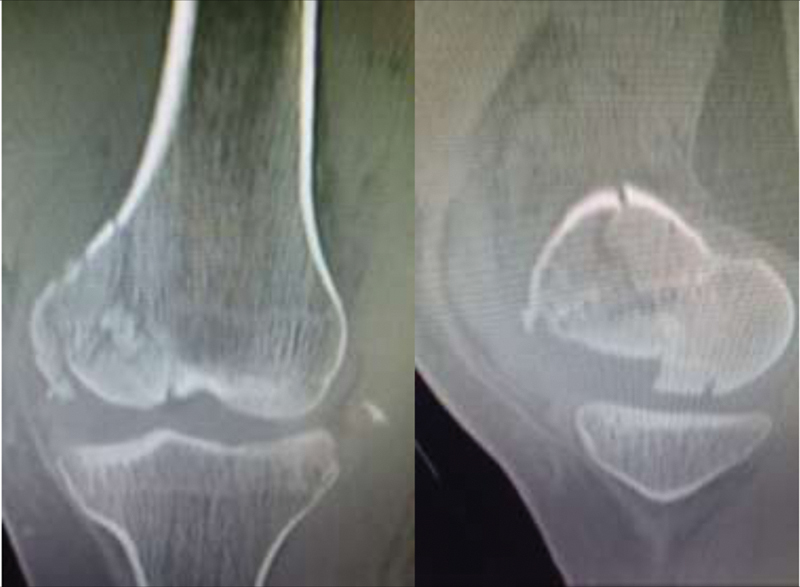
Computed tomography scan—coronal and sagittal cuts.
